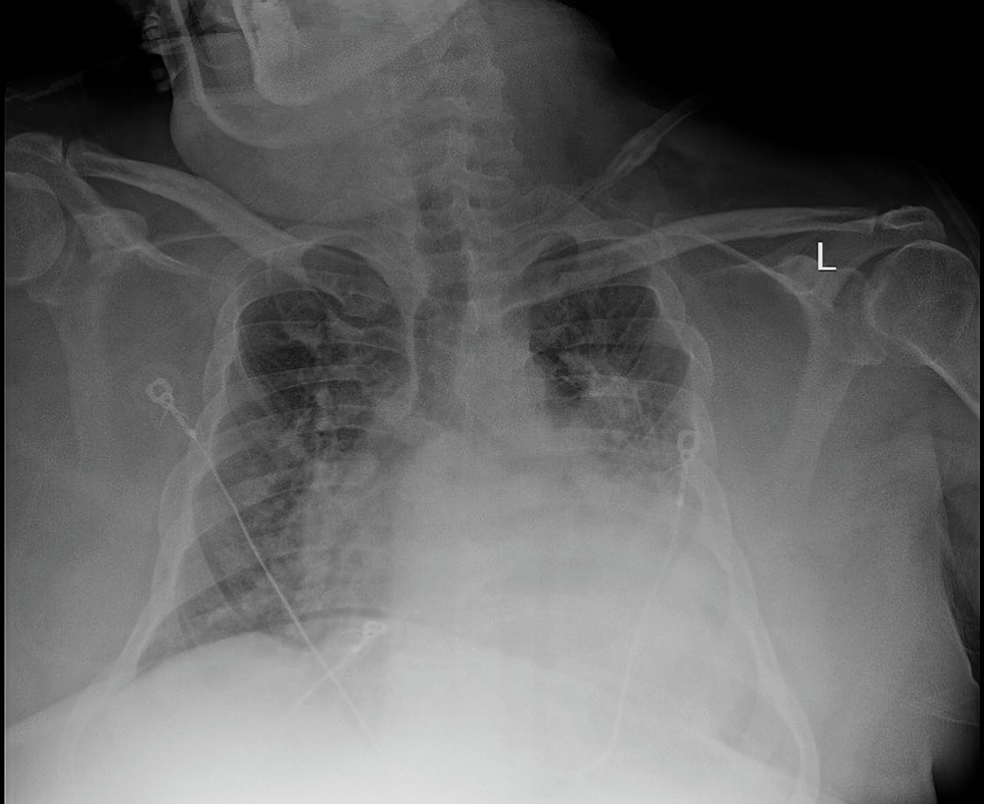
Chest X-ray showing bilateral opacities left greater than right.
